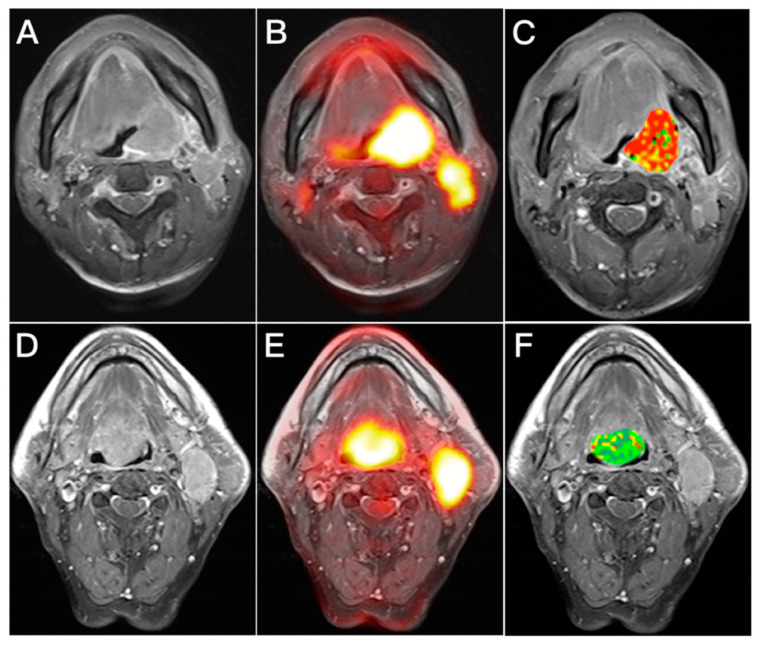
A combination of PET metabolic and IVIM functional biomarkers aided in predicting overall survival. (A–C) Representative axial images of a primary left tonsillar oropharyngeal cancer patient with T3N2b disease. (A) Contrast-enhanced MR image; (B) ^18^F-FDG PET/MRI image; and (C) Contrast-enhanced MR image with an overlaid *D map of the primary tumor. This patient had a high MTV of 111 mL and a high *D value of 481 × 10^−3^ mm^2^/s. This patient died of tumor recurrence with a short overall survival period of 8 months after chemoradiotherapy. (D–F) Axial PET and MRI images of a primary tongue base oropharyngeal carcinoma patient with T3N2b disease. (D) Contrast-enhanced T1-weighted MR image; (E) ^18^F-FDG PET/MRI image; and (F) Contrast-enhanced MR image with an overlaid *D map of the primary tumor. He had a low MTV of 58 mL and a low *D value of 255 × 10^−3^ mm^2^/s. He survived without disease recurrence for 4 years after chemoradiotherapy. MTV: metabolic tumor volume.
